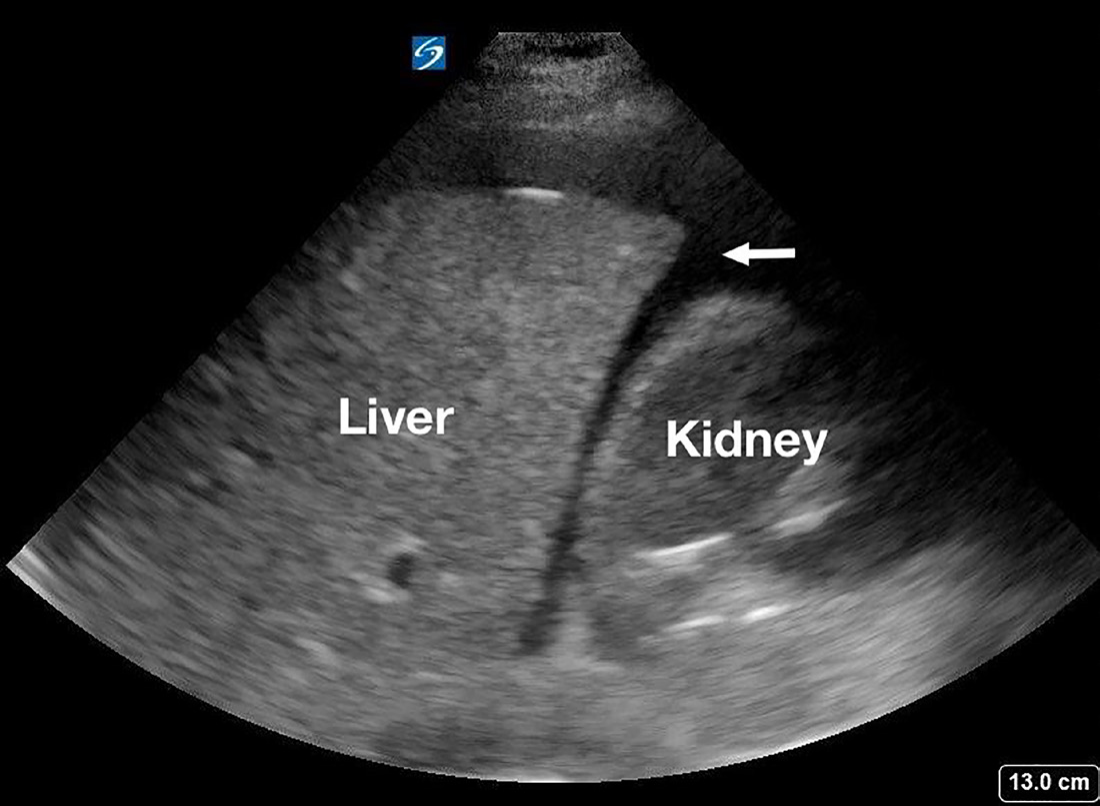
Point-of-care ultrasound of the right upper quadrant of the abdomen demonstrating free fluid in Morison’s pouch (arrow).
